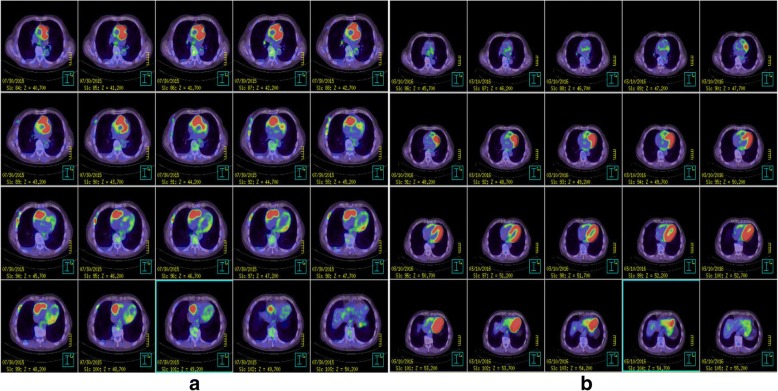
F-18 FDG-PET. a Preoperative PET-CT showed abnormal accumulation in the right atrium surroundings the aortic root. b Postoperative PET-CT showed complete remission after completion of chemotherapy. Accumulation in the left ventricular myocardium was physiological, and the abnormal accumulation around the aortic root and pulmonary artery had disappeared
